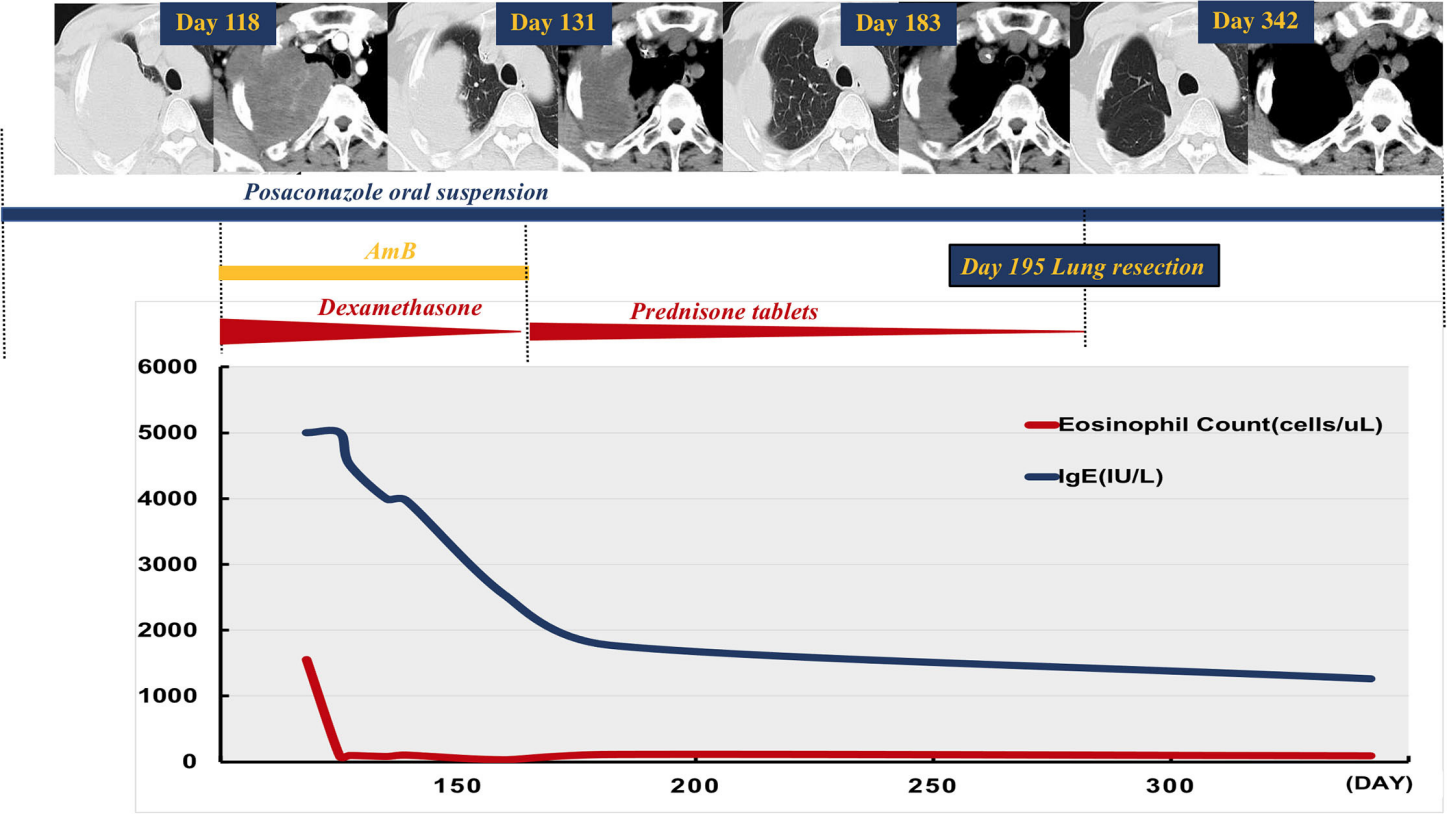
Case time line. Chest computed tomography on day 118 depicted a mass in the right upper lobe of the lung involving the right chest wall, and there was no reduction in its size after 3 months of treatment with oral posaconazole suspension. Concurrent amphotericin B and dexamethasone were started after repeat diagnostic lung biopsy on day 120. Amphotericin B was stopped on day 128. Systemic corticosteroid (SCS, prednisone tablets) was given after repeat imaging on day 131 and depicted a marked reduction in mass size. Radiology on day 183 indicated substantial improvement in the lung lesions with concurrent weaning of SCS. The patient underwent subsequent lobectomy on day 195, and posaconazole was used during the following 3 months. He was well, with no radiological evidence of relapse on day 342 follow-up. Reduction in peripheral eosinophil count and total serum IgE level was observed after initial SCS and anti-fungal therapy.
